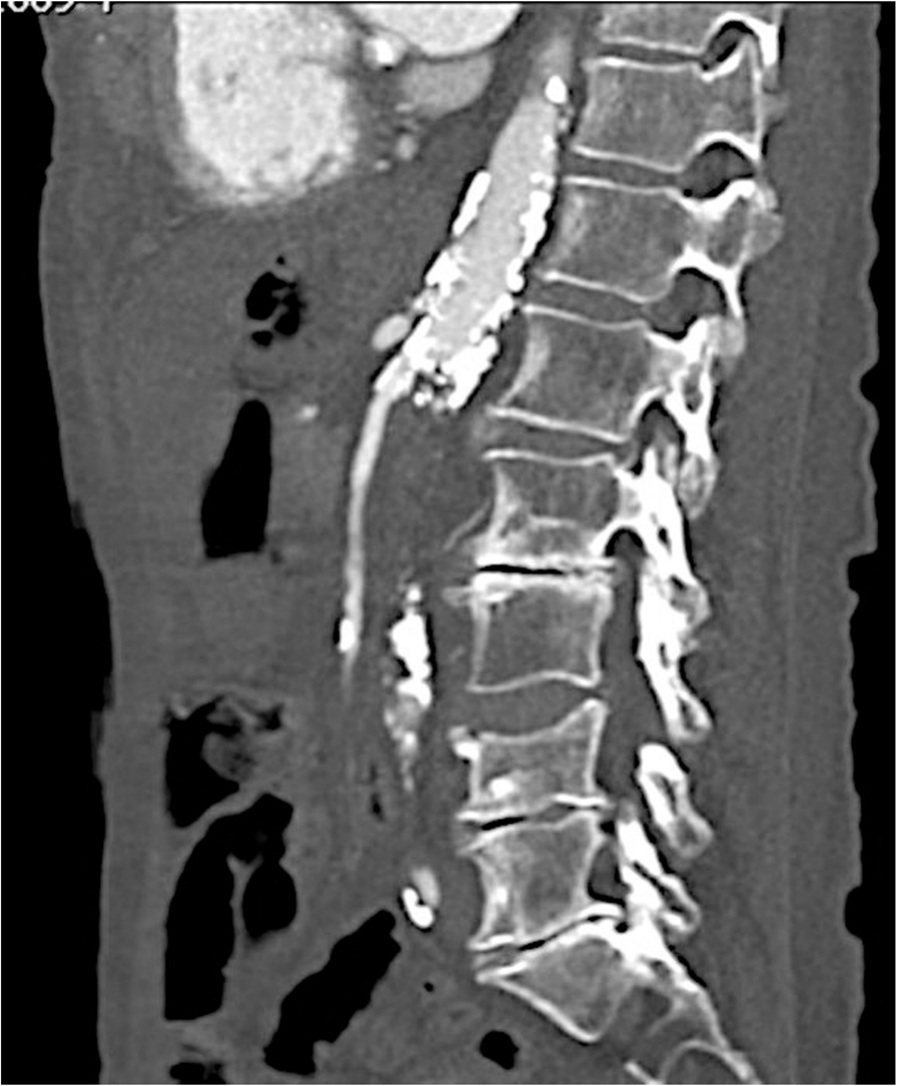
Follow-up CT of the abdomen 13 months after revascularization and stenting of the SMA with free perfusion of the vessel
